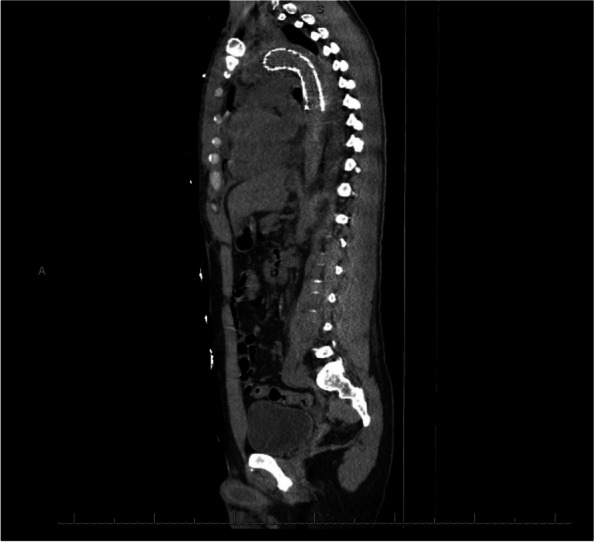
Post-operative day 4 CT angiogram showing positioning of 34 mm x 15 mm Gore thoracic endograft (sagittal cross-section)
